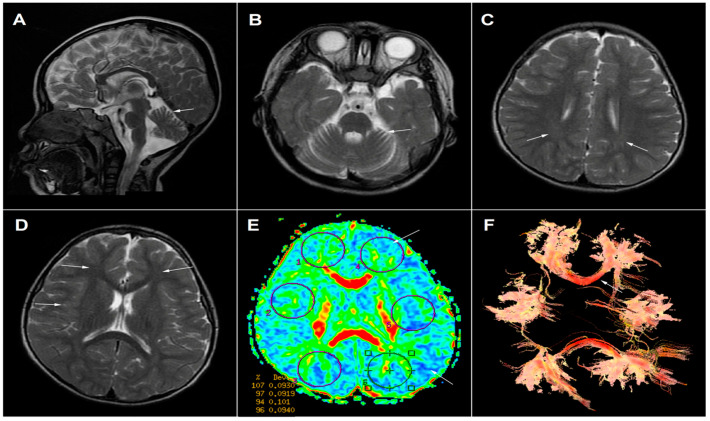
Diffusion tensor-based images of brain MRI. The T2W sagittal (A) and transverse (B) MR images of the brain reveal cerebellar hypoplasia (indicated by white arrow). T2W transverse image demonstrates hypo-intense shadow (white arrow) in the white matter of parietal cortex (C) as well as frontal and temporal cortices (D), indicating poor myelination of the cerebral white matter. Color-coded fractional anisotropy (FA) maps (E) were generated to depict the tracts of nervous system in the brain, with red representing the fibers running transversely from left to right, as well as green and blue respectively representing those running in the posterior-anterior and inferior-superior dimension. (E) reveals a mild FA reduction in left frontal cortex and occipital cortex (white arrow), and a thinning of the corpus callosum fibers within left minor forceps compared to the right part. (F) is a fiber tractography demonstrating that the fiber tracts originating from the corpus callosum show a random distribution and a decrease in the numbers and extension of the fibers (white arrow). Meanwhile, it also reveals a thinning of the corpus callosum fibers within left minor forceps when compared with the right part (white arrow), which is in consistent with the findings in (E).
